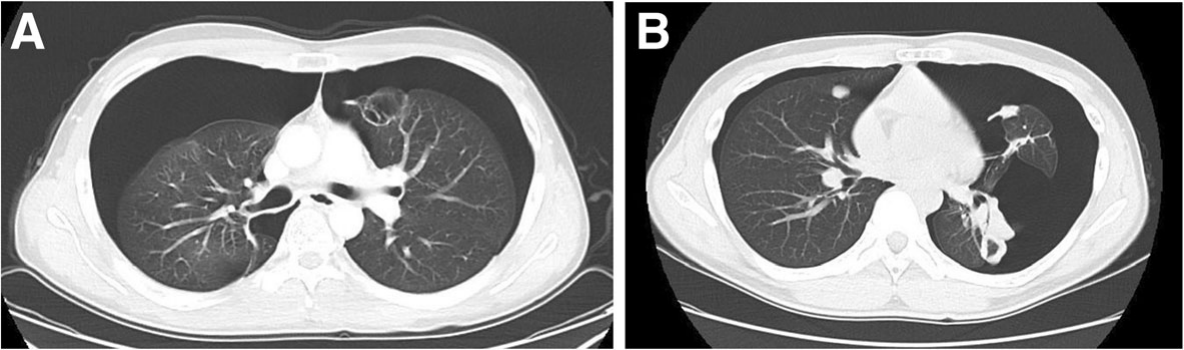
Radiographs of two bilateral pneumothorax events. a A bilateral pneumothorax in Patient 3 on treatment day 61. In this patient, cavitations of lung lesions were observed. b Bilateral pneumothorax events of Patient 4 on treatment day 95. In the CT scan, progression of lung lesions was also observed
